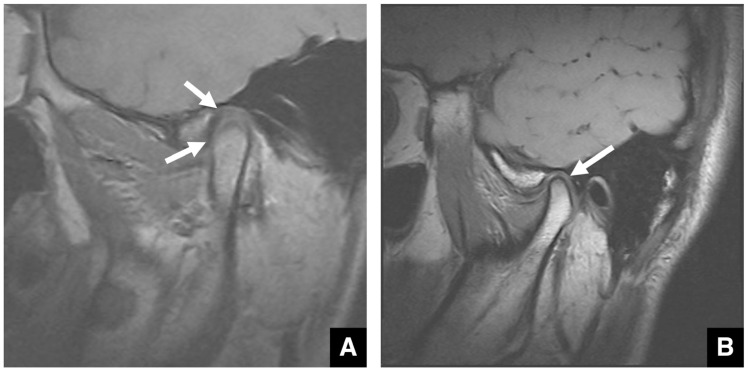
DCLS as an indicator of normal mandibular condyle development. (A) MR image (TR 1050 ms, TE 18 ms, slice thickness 3.0 mm) shows DCLS in the normal temporomandibular joint of a 10-year-old girl (arrows). (B) DCLS is not observed in an MR image of the normal temporomandibular joint in a 36-year-old man (arrow). Permission has been obtained to publish all figures.
